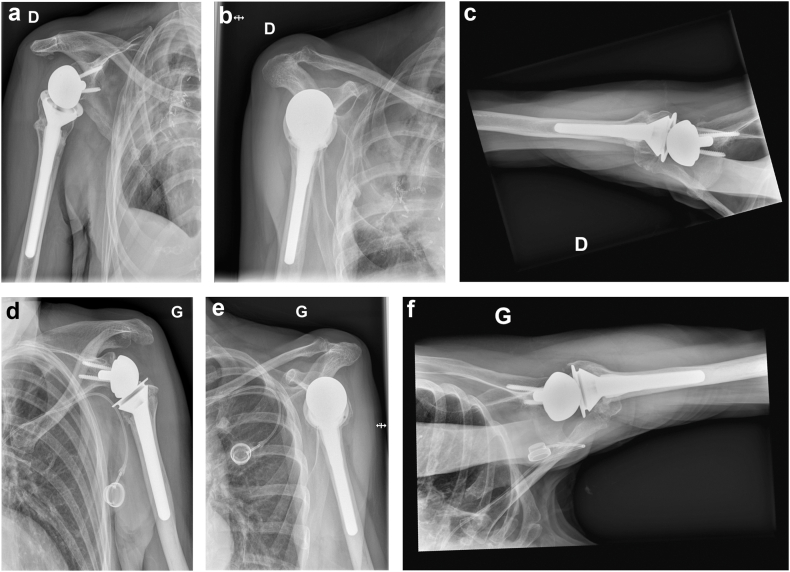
X-ray (anteroposterior, Neer, axial views) 6 months postoperative of Right (a-c), shoulder and Left (d-f) shoulder, showing correct positioning of reverse total shoulder arthroplasty and healed tuberosities on both sides.
